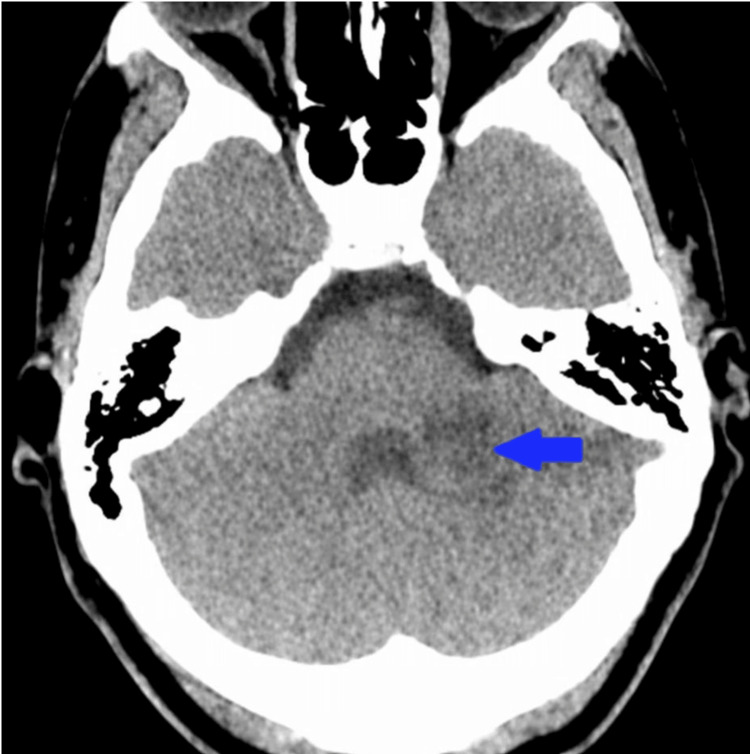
Noncontrast axial CT of the brain demonstrating left cerebellar infarction. Initial noncontrast head CT shows an approximately 3 cm hypodense lesion in the left cerebellar hemisphere, consistent with acute to subacute infarction, without hydrocephalus or significant mass effect.
